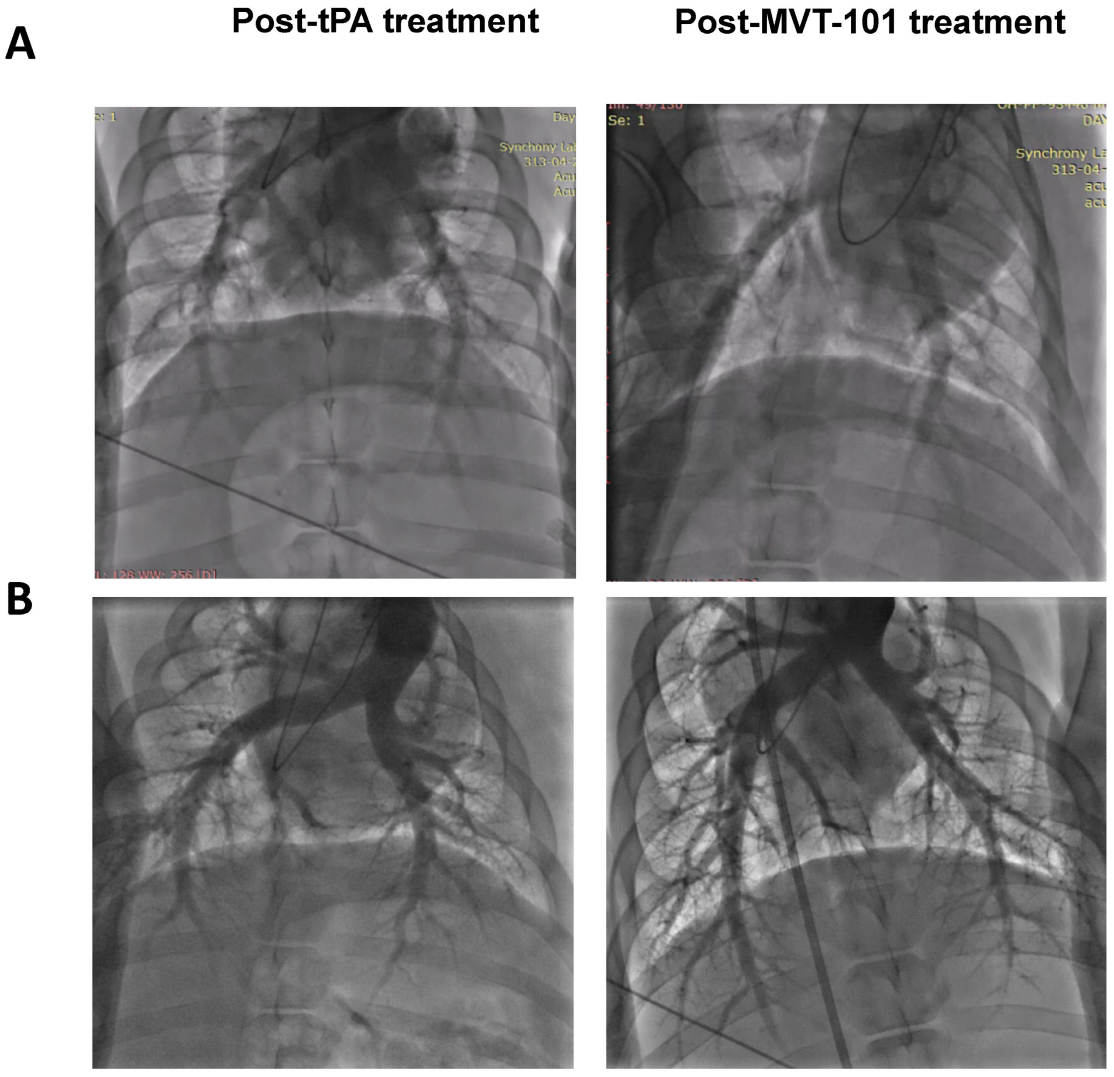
Pulmonary angiograms of post-tPA treatment (left) and post-MVT-101 treatment (right) with Endovascular Ultrasound (Panel A) or Transcutaneous Ultrasound (Panel B).
